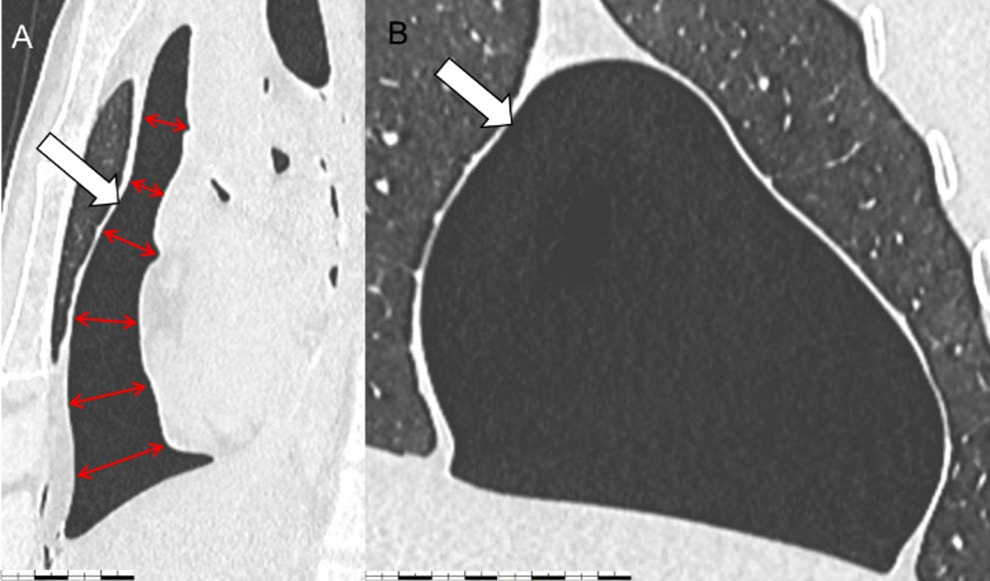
CT scans of the pericardium (sagittal section A and frontal section B) the white arrow indicates the pneumopericardium, while the red double-headed arrows highlight the flattened heart
